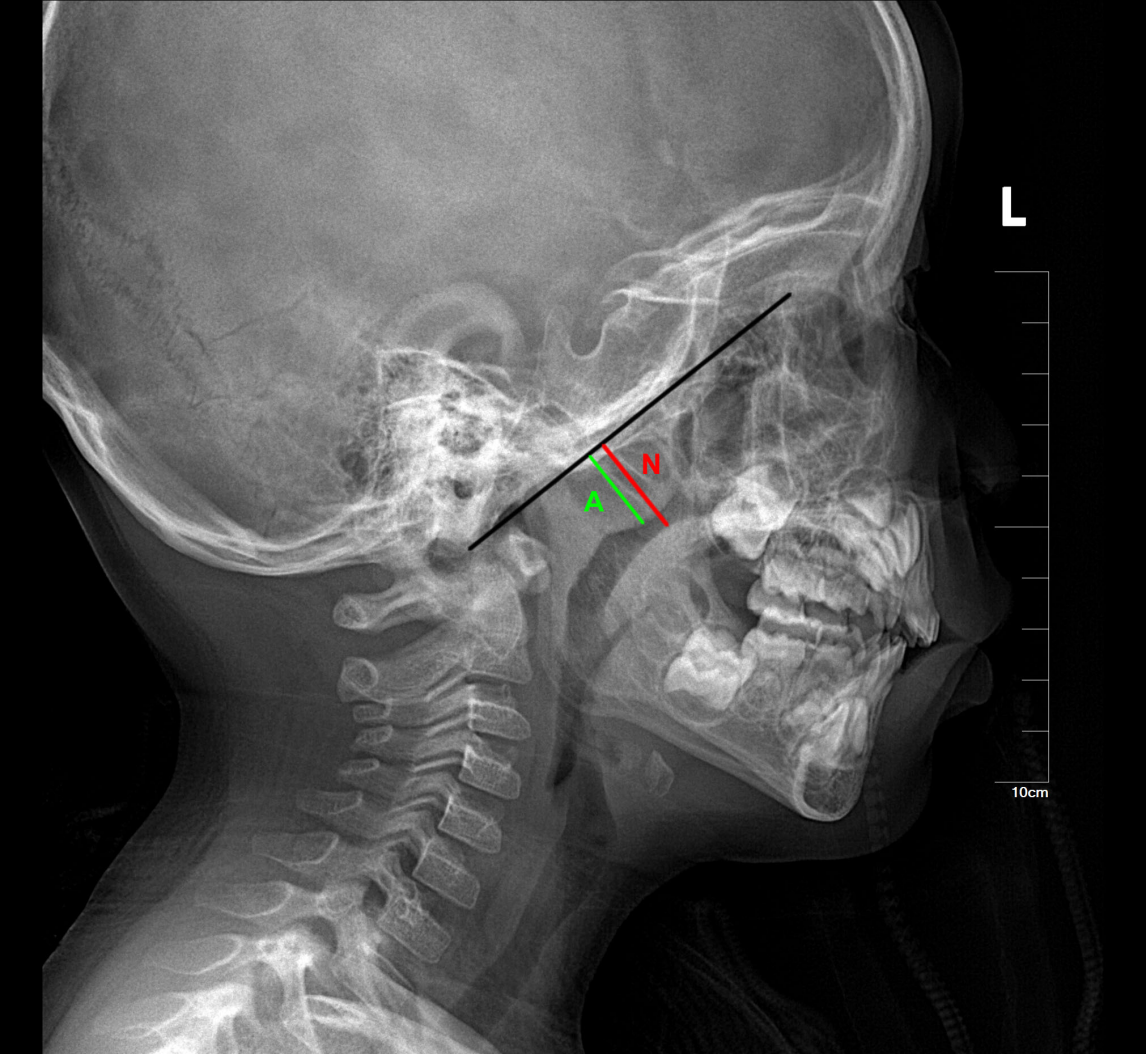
The Fujioka method for measuring adenoid size on lateral radiographs by calculating the adenoid/nasopharyngeal (A/N) ratio. The black line marks the lateral contour of the occipital slope of the skull on the lateral radiograph. The green line (A) represents the vertical distance from the most prominent point of the adenoid to the lateral contour of the occipital slope of the skull. The red line (N) represents the width of the nasopharyngeal cavity at the level of the most prominent point of the adenoid.
